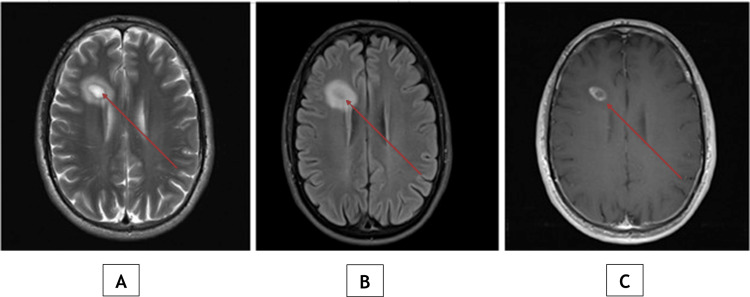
Contrast-enhanced brain MRI showing a ring-enhancing right frontal lesion with surrounding edema Axial T1-weighted post-contrast MRI (C) shows an irregular ring-enhancing lesion in the right frontal lobe (arrow) with heterogeneous peripheral enhancement and focally thicker margins. Surrounding vasogenic edema is seen as hyperintensity on T2-weighted (A) and fluid-attenuated inversion recovery (FLAIR) (B) sequences.
